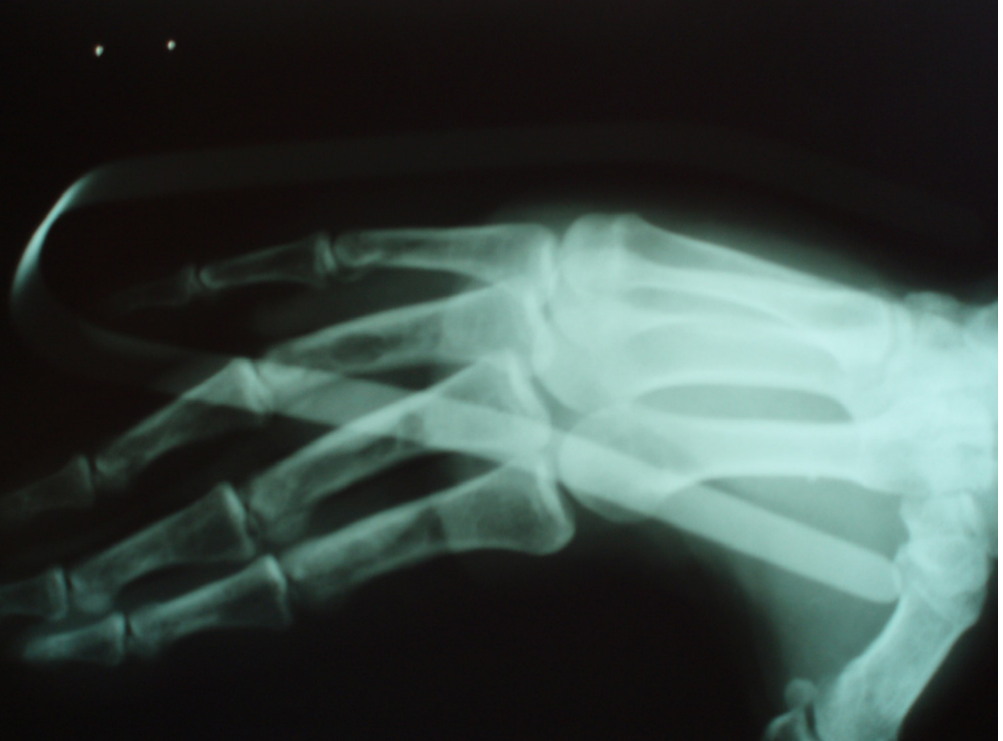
Lateral roentgenogram after surgery.
